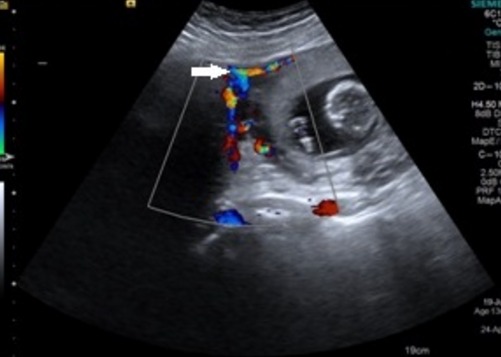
Ultrsonographic picture showing bridging vessel (white arrow) between the mass lesion and gravid uterus
